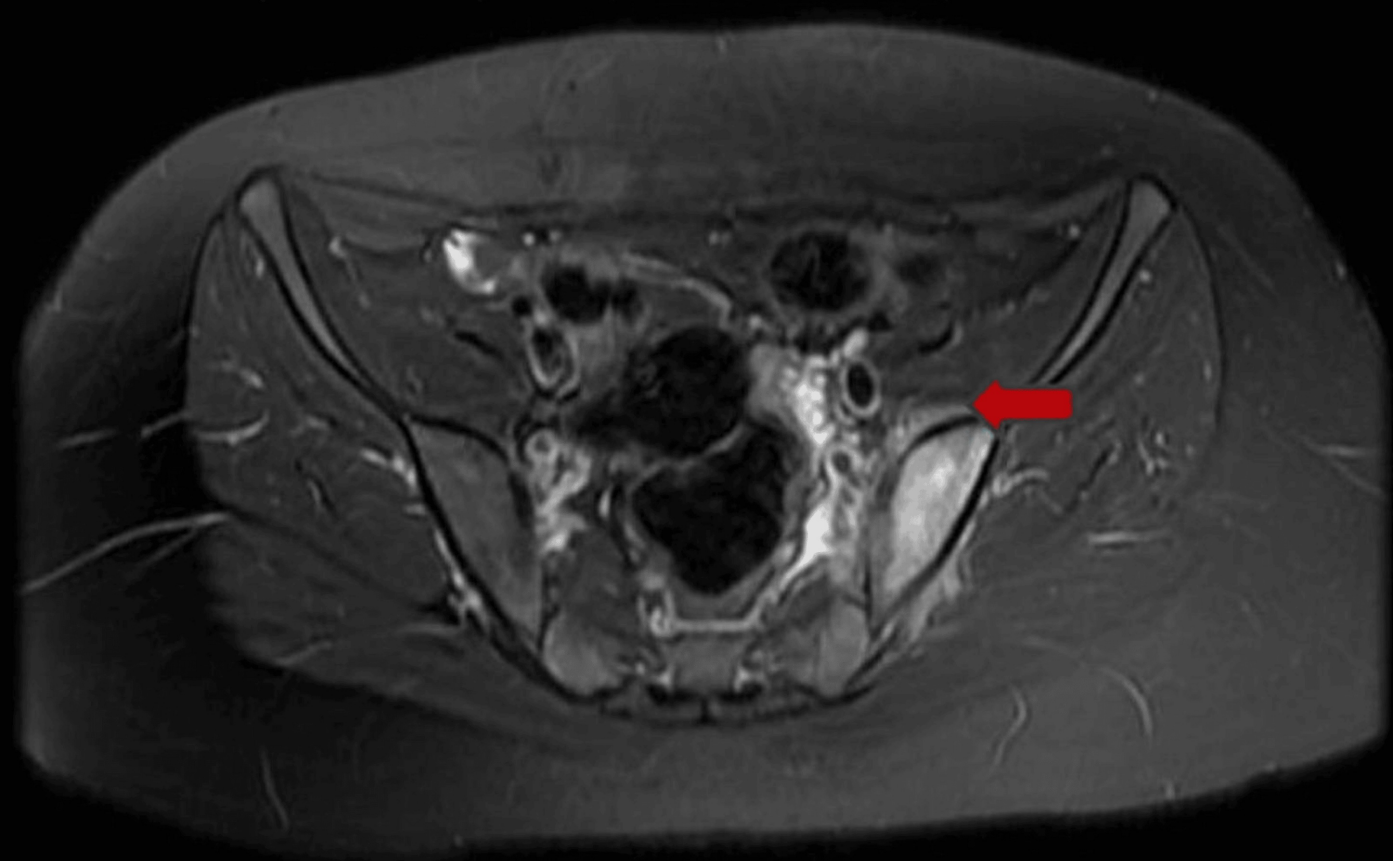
Follow-up hip MRI (day 72) with residual left sacral and iliac marrow edema
